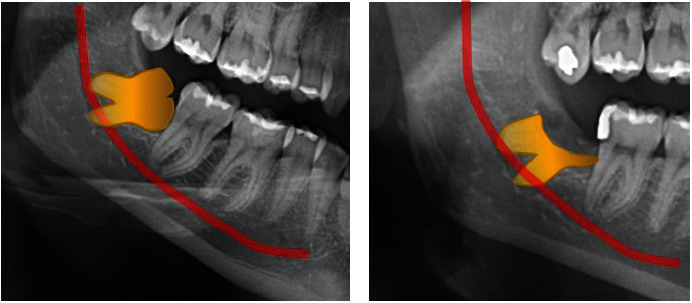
Clinical case in which a M3 coronectomy was performed, due to the very close relationships between M3 roots and IAN: (a) radiographic image before surgery and (b) postsurgery radiographic image.
